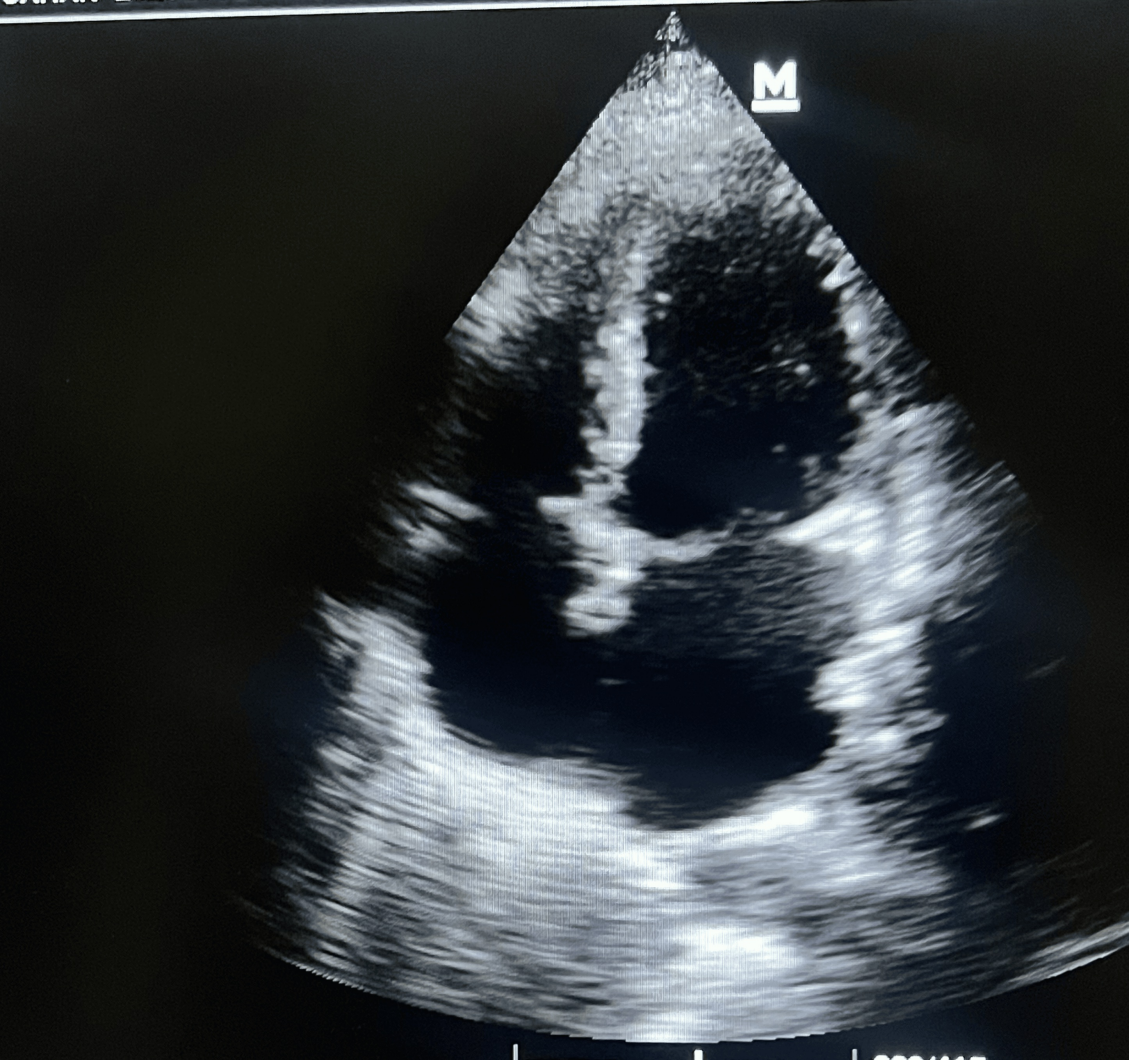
Admission 2D echo The initial 2D echocardiogram shows severe biventricular dysfunction and global hypokinesia.
